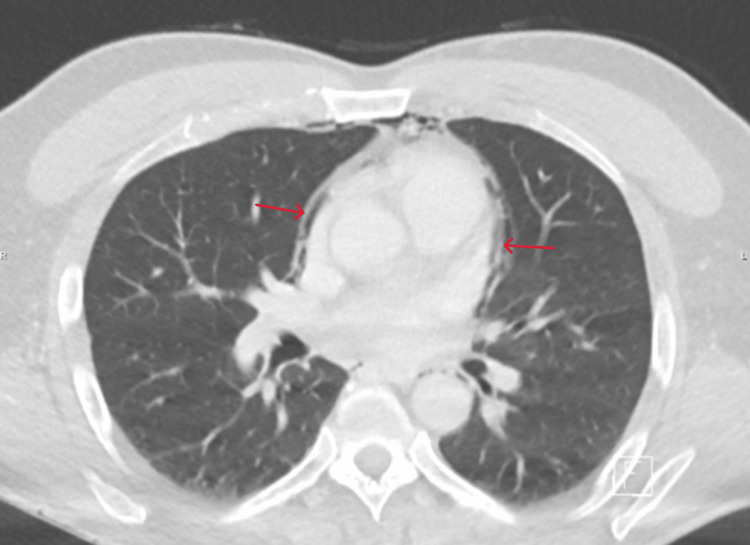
CT scan showing pneumopericardium CT: computed tomography
